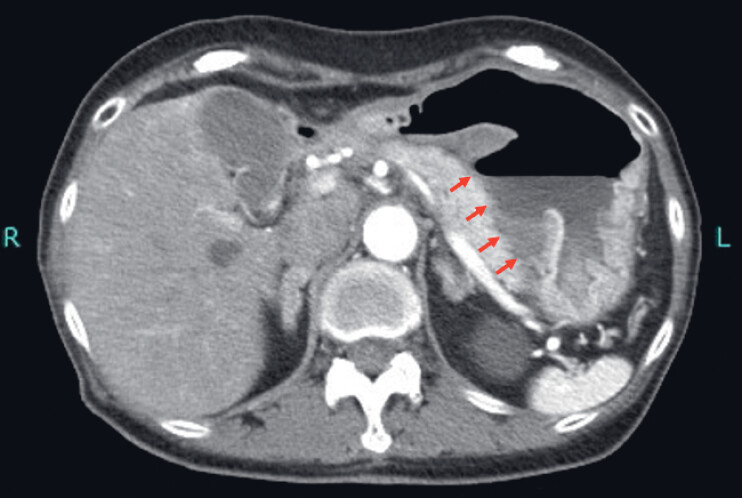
Computed tomography revealed a gastric ulcer penetrating into the pancreas (red arrow) with no free air within the abdominal cavity.
